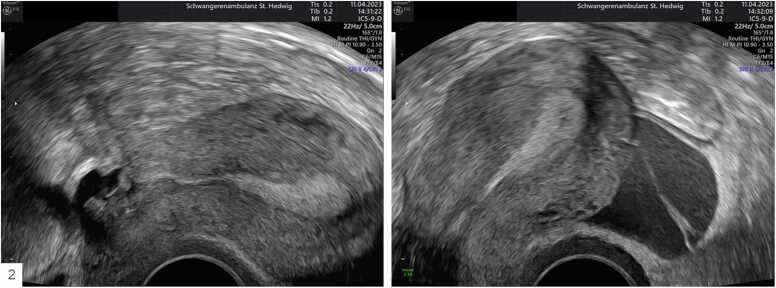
Left: Anterior uterine wall defect resulting in retroflexio uteri Right: Sonographically visible retrograde menstruation.
